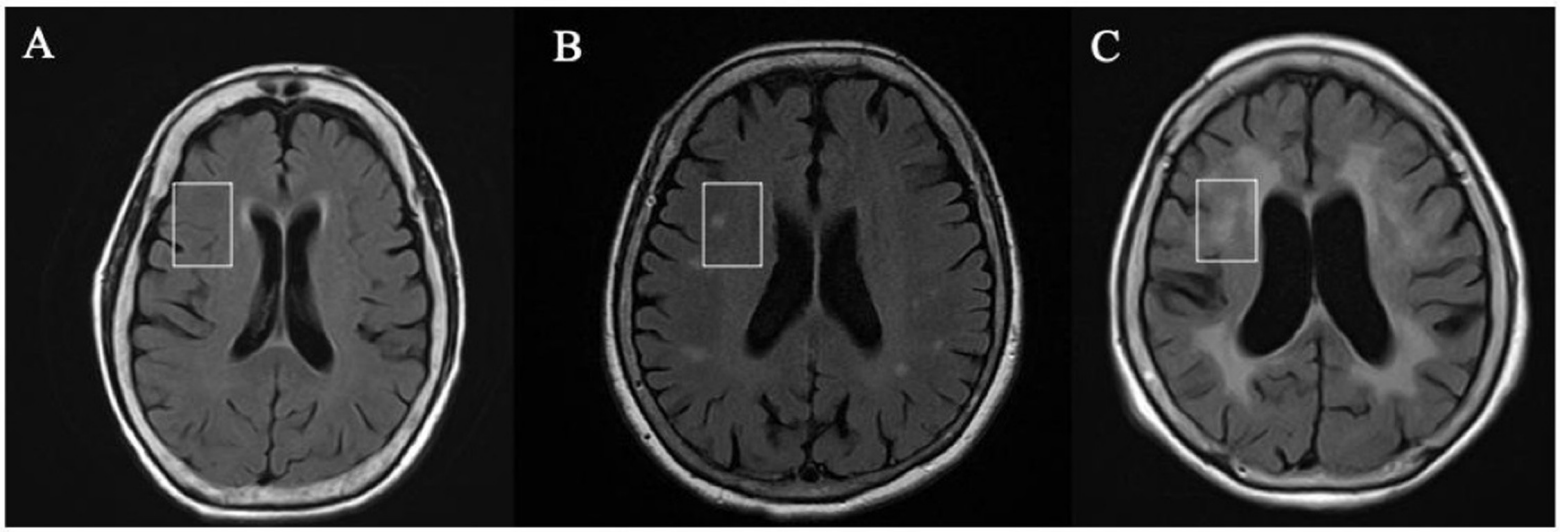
Brain MRI images of WMH severities corresponding to CHIPS scores as circumscribed by white rectangles. (A) Absence of WMHs (CHIPS score: 0) (B) Mild WMHs (CHIPS score: 1), as indicated by the WMHs occupying less than 50% of the marked area (C) Moderate-to-severe WMHs (CHIPS score: 2), as indicated by the WMHs occupying more than 50% of the marked area Note: WHM white matter hyperintensities, CHIPS: Cholinergic Pathway Hyperintensity Scale.
